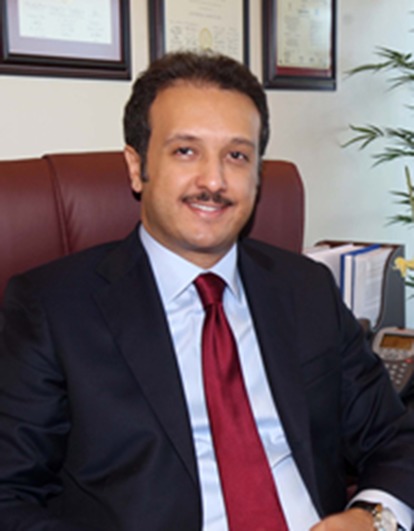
Dr Mohammed Al Jarallah is currently the head of cardiology at Sabah al Ahmed cardiac center, Kuwait. He obtained his M.B.Bch form the faculty of medicine, Kuwait University in 1999. Then, he went to do his Internal medicine training at McGill University, Quebec, Canada from the year 2001 to 2004. During which he was certified with the American board of internal medicine in 2004 and became a fellow of the Royal College of Physicians of Canada for internal medicine in 2005. He did his cardiology fellowship at McGill University from 2004 to 2007 followed by a year of fellowship in echocardiography. He was certified with the American board of cardiovascular diseases and became a fellow of the royal college of physicians of Canada for cardiology in 2007.
